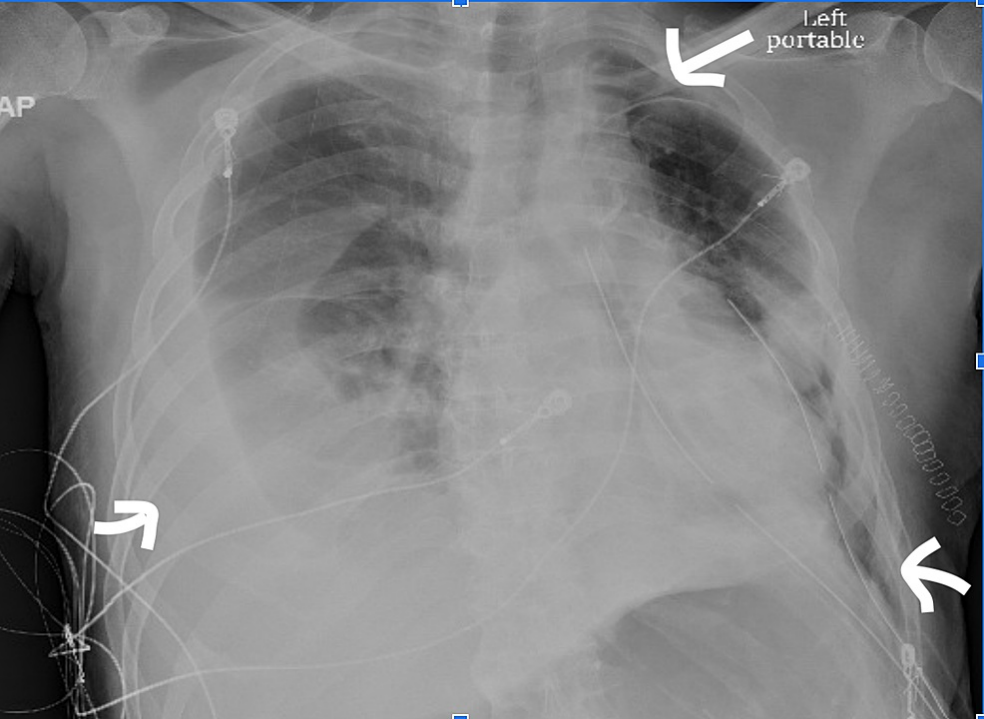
Chest X-ray after thoracotomy with two left-sided chest tubes and a small-to-moderate left pneumothorax (indicated by arrows on the right side of the X-ray) with a persistent large right pleural effusion (arrow on the left)
